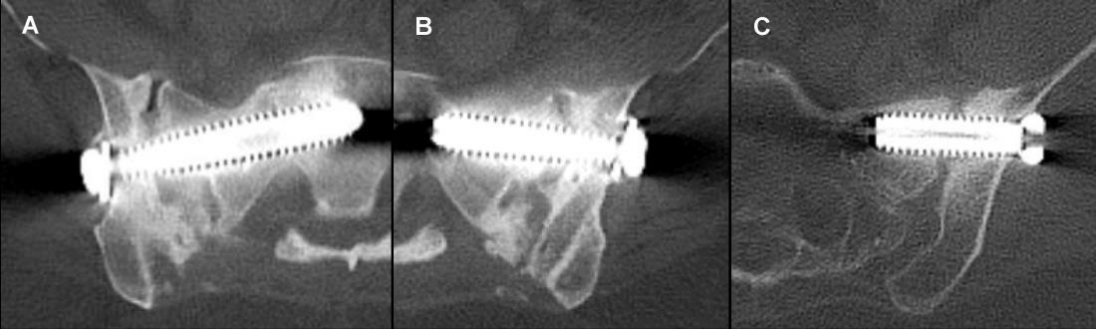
CT images assessed as bridging bone with solid fusion (A), bridging bone with possible fusion (B) and not fused (C).
